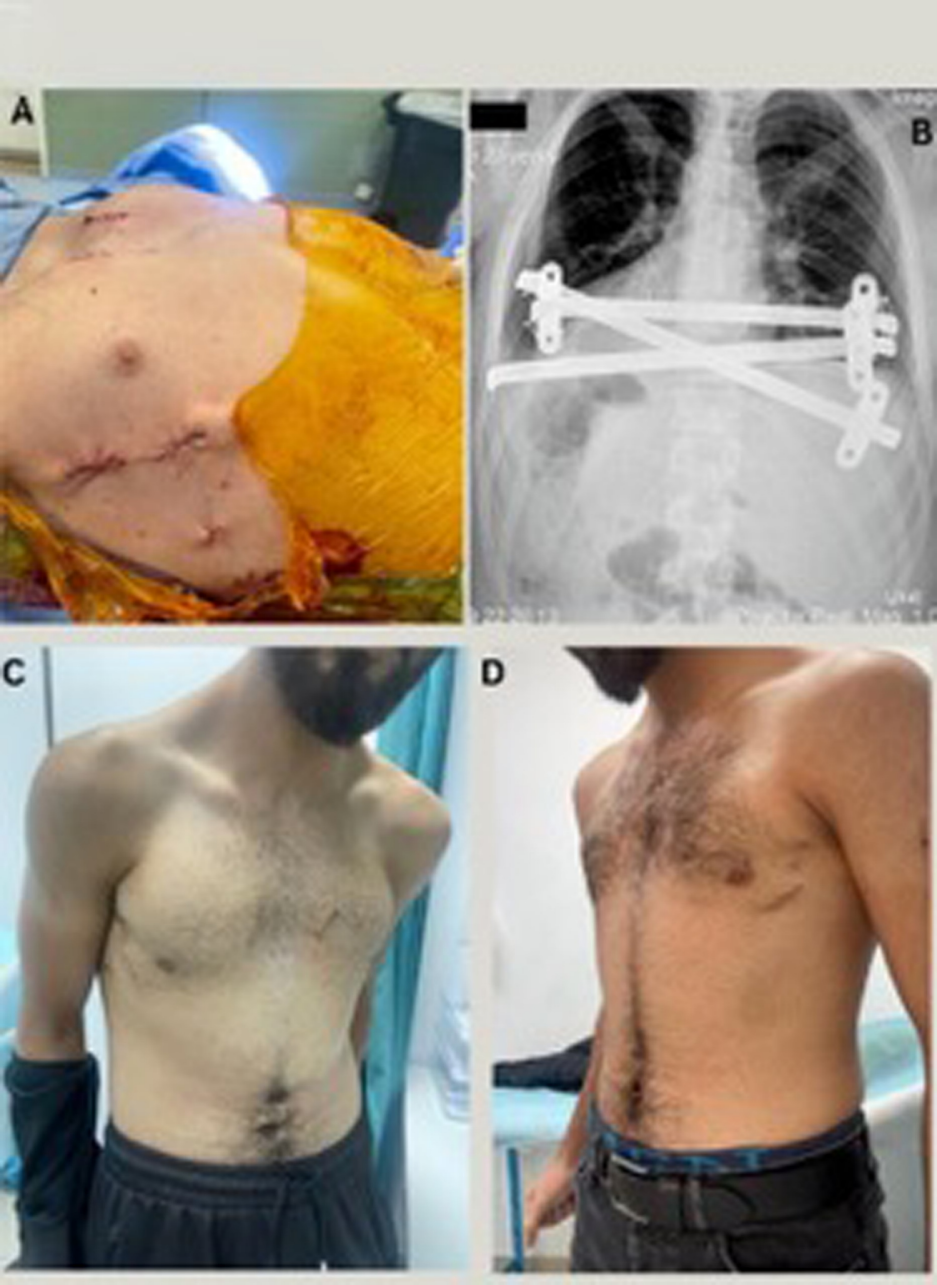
(A) Supplementary. Post operative | case 1. (B) Supplementary. Post operative x-ray | case 1. (C) Supplementary. 30 days postoperative lateral view | case 1. (D) Supplementary. 30 days postoperative anterior view | case 1.
